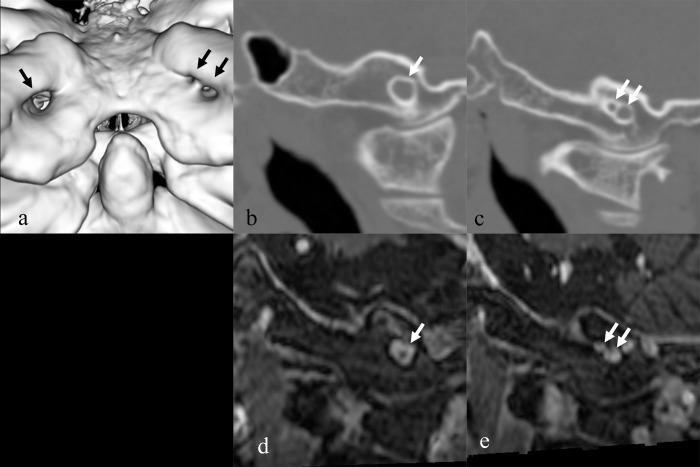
46-year-old female with meningioma had left Double hypoglossal canal. (a) 3D CT image (b) Oblique sagittal reconstructed CT image showed the right hypoglossal canal. (c) Oblique sagittal reconstructed CT image showed left double hypoglossal canal. (d) Low signal intensity of the right hypoglossal nerve within an enhancing venous plexus was seen on oblique sagittal reconstructed image of contrast enhanced and fat saturation T1WI. (e) Low signal intensity of left hypoglossal nerve within a duplicated enhancing venous plexus was seen on oblique sagittal reconstructed image of contrast enhanced and fat saturation T1WI.
